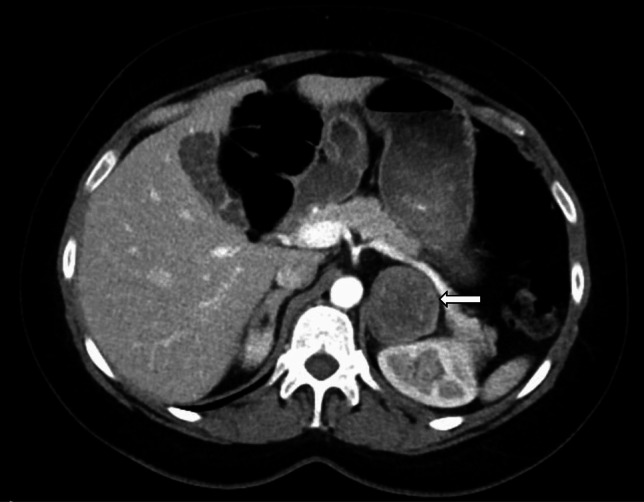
CT scan showing a left adrenal mass
